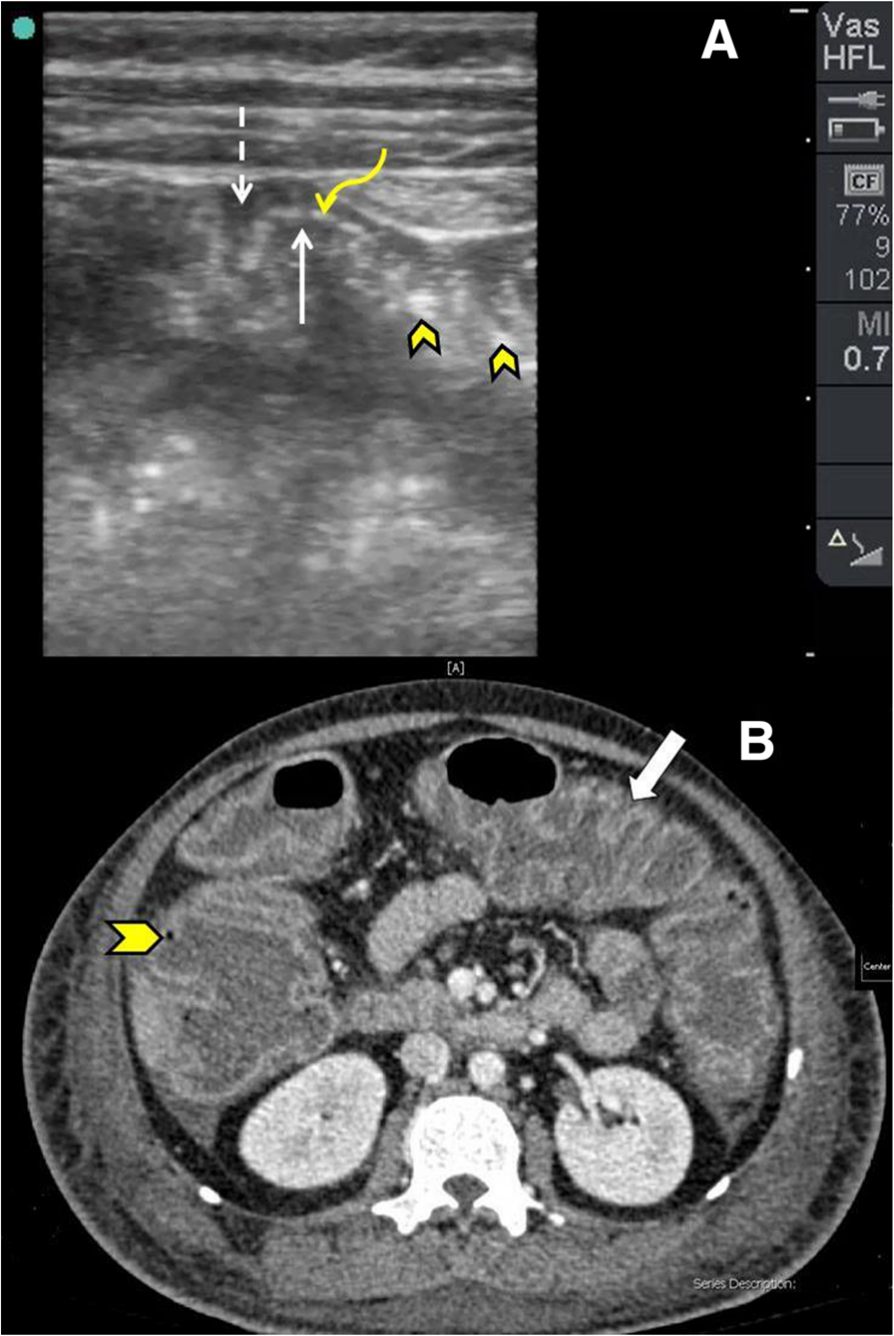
POCUS study (a) using a high-frequency linear probe in multi-trauma patients who developed diarrhoea 2 weeks after starting antibiotic administration. The colonic wall is thickened with three distinct layers, mucosa (white arrow), submucosa (curved yellow arrow), and muscularis propria (interrupted arrow). Hyperechoic lines are seen covering the mucosa (arrowheads). These can be either pseudomembranes or air bubbles. These findings give the classical accordion sign of pseudomembranous colitis. Abdominal CT scan with intravenous contrast (b) which was performed at the same time shows the same accordion sign (white arrow). CT scan has the advantage of showing the hyperaemic mucosa with air bubble in the colonic wall (arrowhead)
